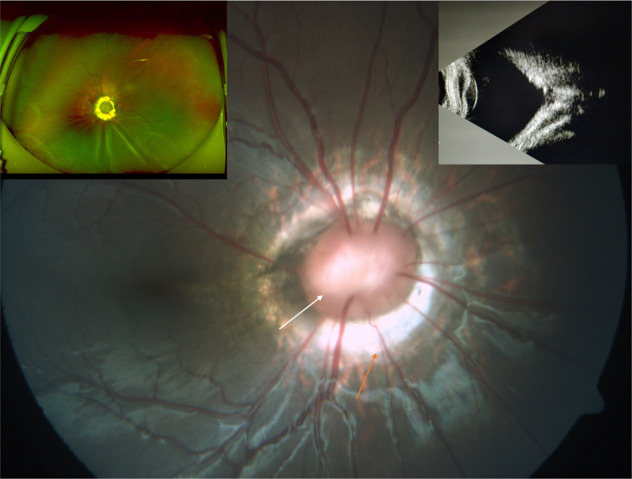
Morning glory syndrome. (i) Typical appearance of the optic disc with tuft of glial tissue at centre (white arrow), peripapillary RPE atrophy (orange arrow) and relatively straight retinal blood vessels radiating from the optic disc. (ii) Left inset shows a case of retinal detachment in an eye with morning glory syndrome. Observe the radiating folds caused by the puckering of the retina towards the disc. (iii) Right inset shows picture of ultrasonography demonstrating the funnel-shaped posterior excavation.
